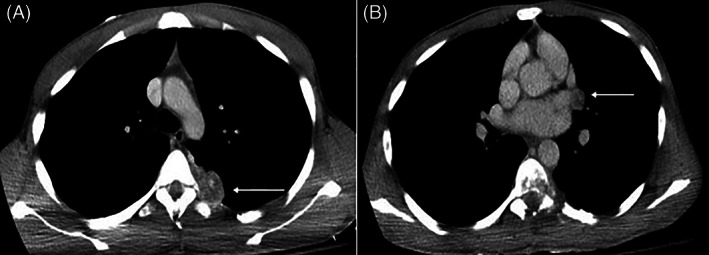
Eleven‐month follow‐up axial contrast enhanced chest CT mediastinal window at T7 level (A); white arrow demonstrates interval progression of residual tumor and left perihilar lymphadenopathy (B)
